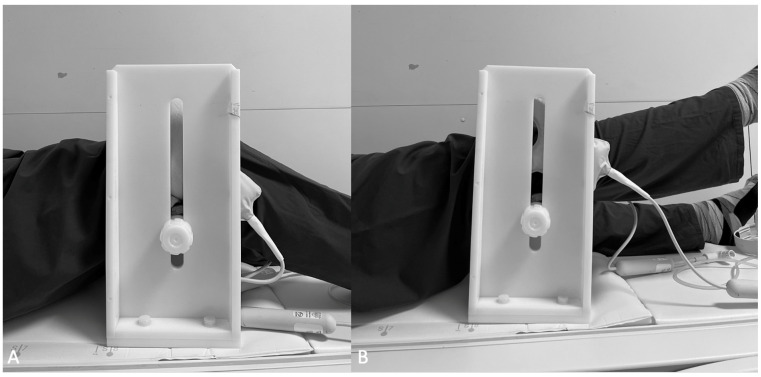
Examination set-up for dynamic magnetic resonance imaging (MRI). The patient’s knees were stored in 40° of flexion, using a customized positioning device (A). Two ring coils (Philip, Best, The Netherlands) were placed on either side of the knee. During image acquisition, the knees were actively moved between 40° and full extension (B).
